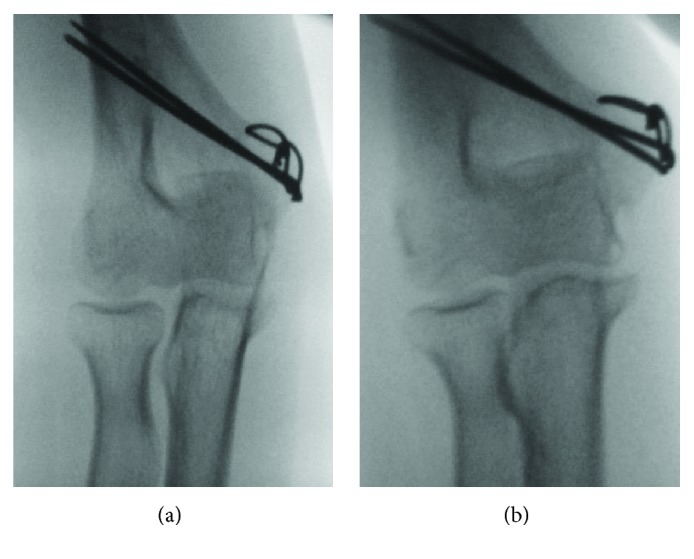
Postoperative stress X-ray of the right elbow evaluated under anesthesia (4 months after the primary surgery). There was no difference of the instability.
